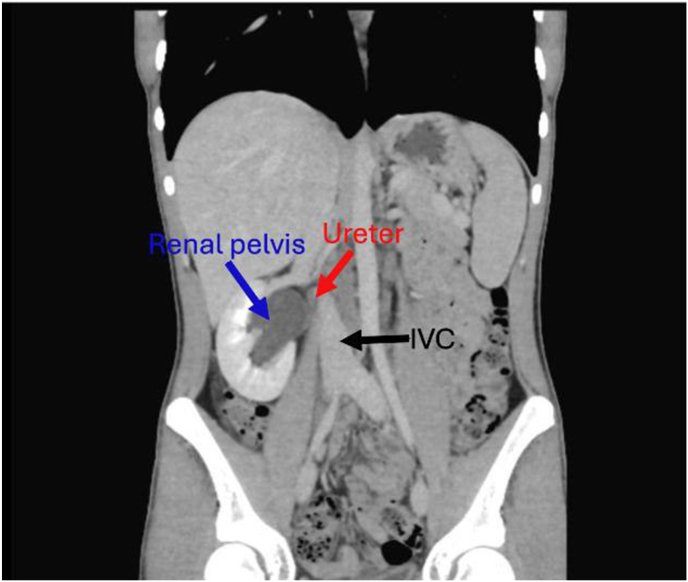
Renal CT scan with contrast.
